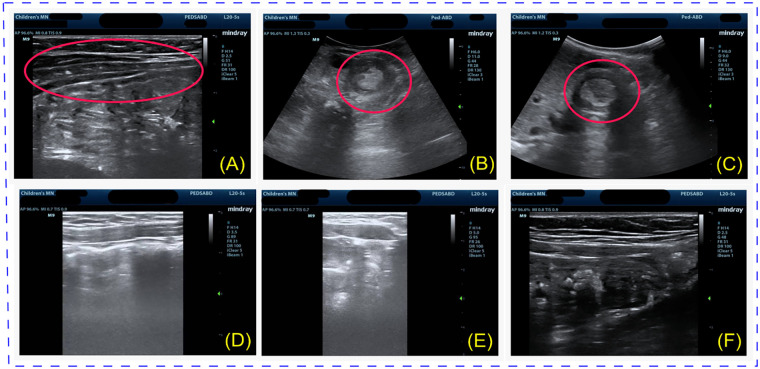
The first row demonstrates point-of-care ultrasound (POCUS) images for intussusception, and the second row demonstrates POCUS images for normal persons. Images A and F demonstrate the longitudinal view, and other images represent the cross-sectional view.
